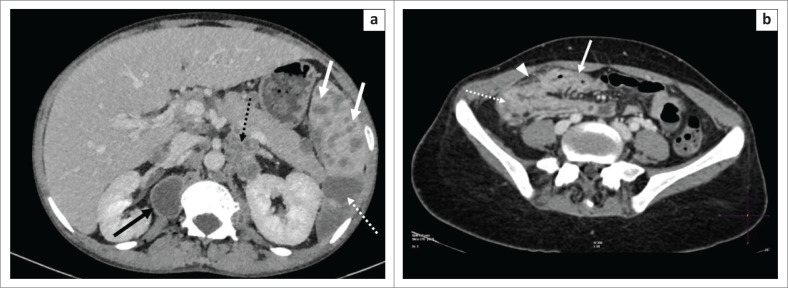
(a) A 34-year-old human immunodeficiency virus-positive female, on retroviral treatment, with previously treated multidrug-resistant tuberculosis presented with acute on chronic gastroenteritis and loss of weight. Axial post-contrasted computed tomography through the upper abdomen revealed multiple splenic micro- (solid white arrows) and macro- (white arrow with dashed line) abscesses with enlarged necrotic right paraspinal (solid black arrow) and clustered necrotic left para-aortic lymph nodes. (b) Axial slices through the pelvis of the same patient revealed diffuse, irregular thickening and enhancement of the terminal ileum (solid white arrow) and caecum (white arrow with dotted line) with peri-caecal fat stranding (white solid arrow head).
